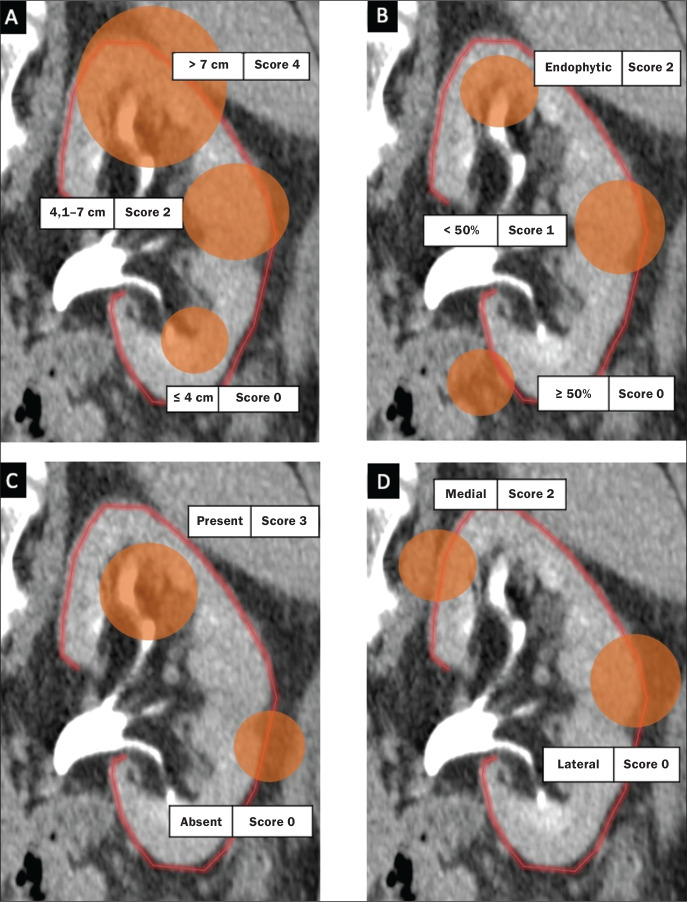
Reconstruction of a sagittal CT scan, showing the tumor features used in order to calculate the SPARE score. A: Tumor size. B: Exophytic proportion. C: Renal sinus involvement. D: Tumor location.
